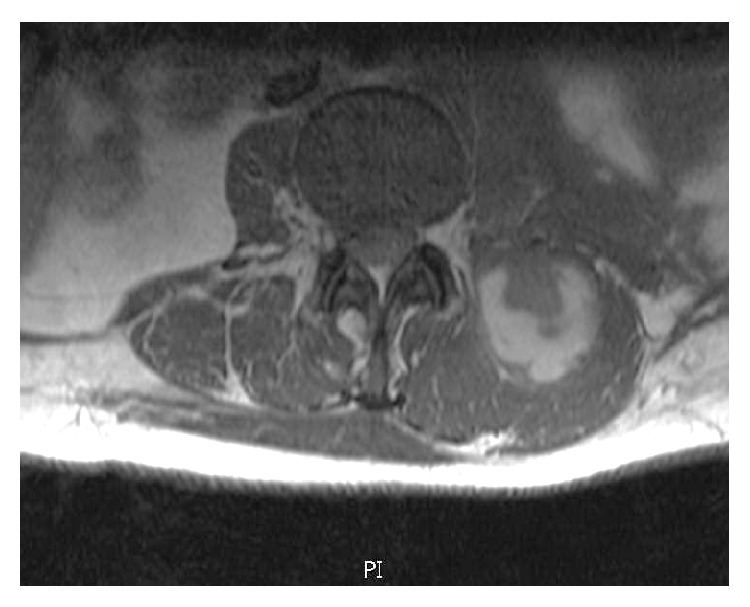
Axial section of MRI at the level of L5 showing left paraspinal intramuscular hematoma.
